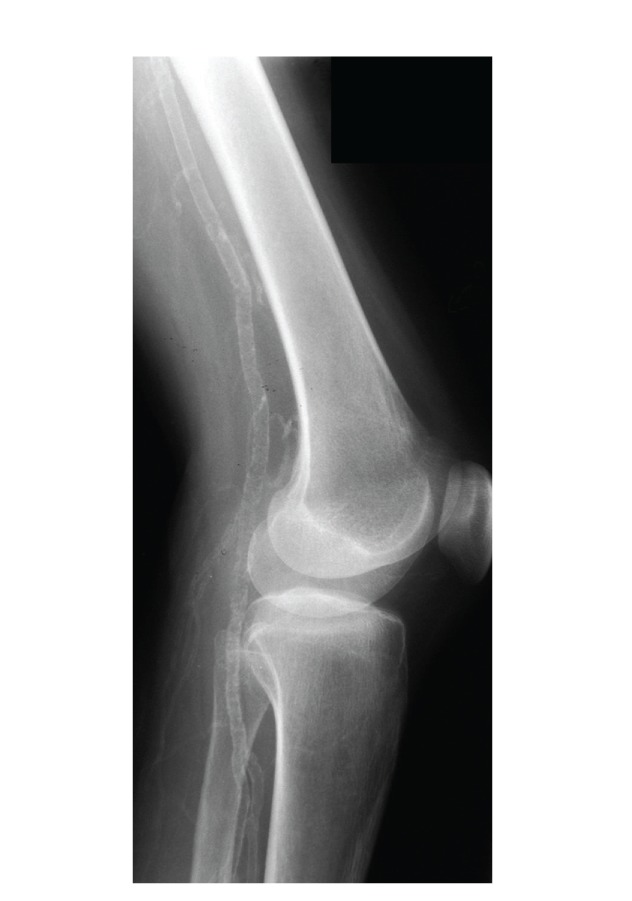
Plain radiograph of right leg showing diffuse vascular calcification of the femoral and popliteal arteries.
